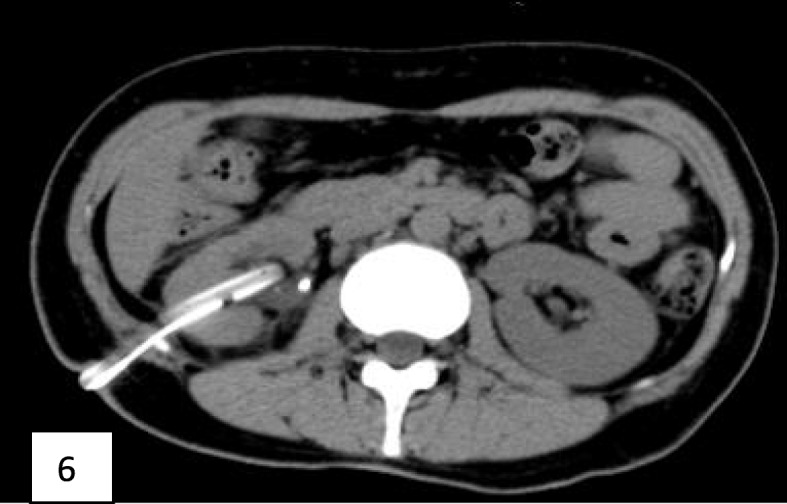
Shows the repeat non-contrast CT scan after the second percutaneous nephroscopy
